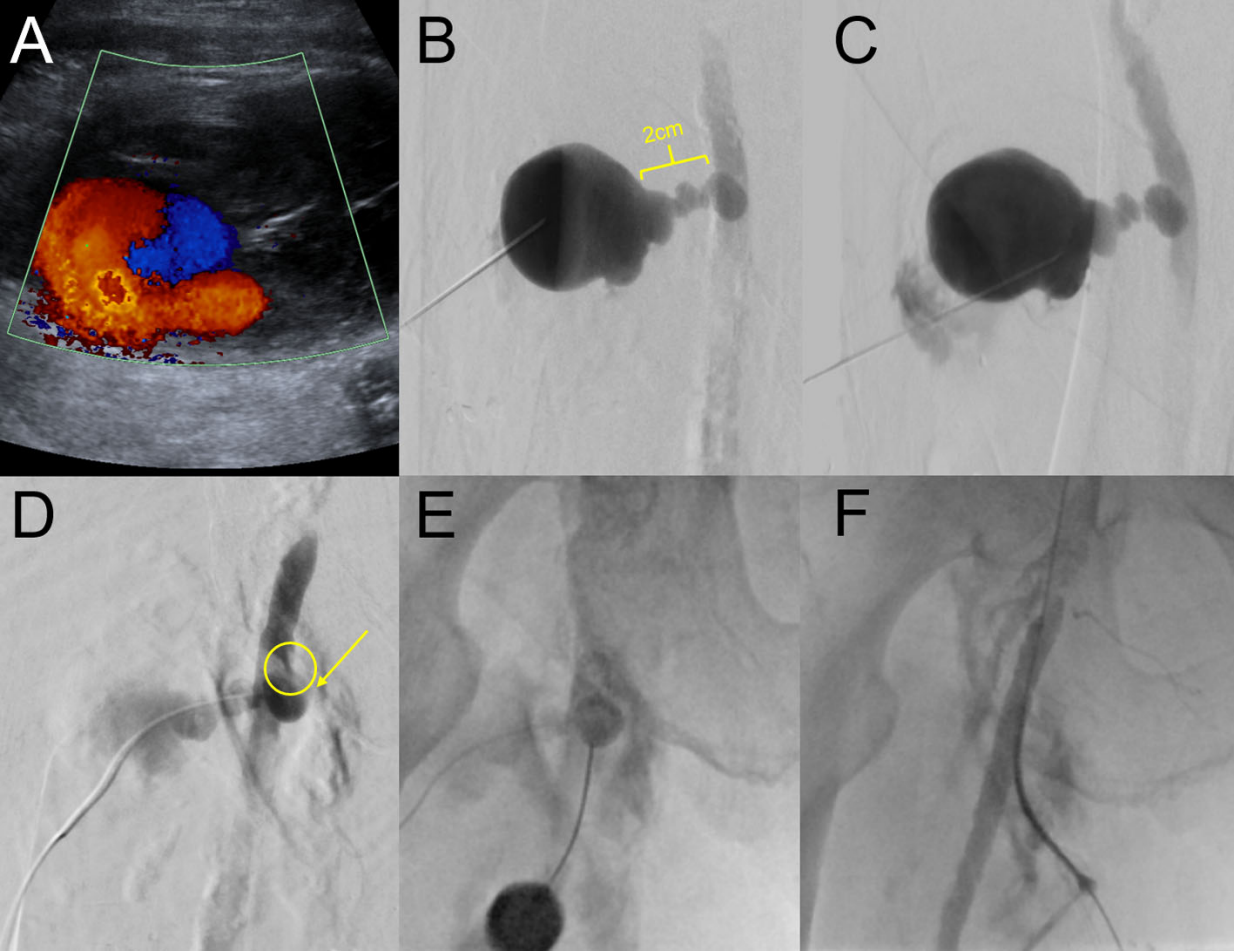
Gigantic PSA in a 75-year-old patient (Patient No. 4) after TAVI, which was successfully closed by the TA maneuver 6 days after the intervention. A Sonography of the gigantic PSA in the right groin. B Initial puncture far away from the PSA neck without any chances to probe the neck via a microwire. C Second puncture closer to the multilobulated neck but still without any realistic chances to reach the neck and the pelvic axis. D Nevertheless, via the second puncture, it was possible to position a MAK mini-sheath close to the neck. E Parallel fluoroscopic puncture and visualization of the PSA via the mini-sheath while rotating the tube made it possible to directly puncture into the neck. F Next, a 5F sheath was inserted successfully, and the TA maneuver could be performed. Although the PSA was gigantic in size, its small and lobulated neck promised good chances of success. Successful closure was confirmed by complete absence of perfusion from the PSA in sonography the day after
